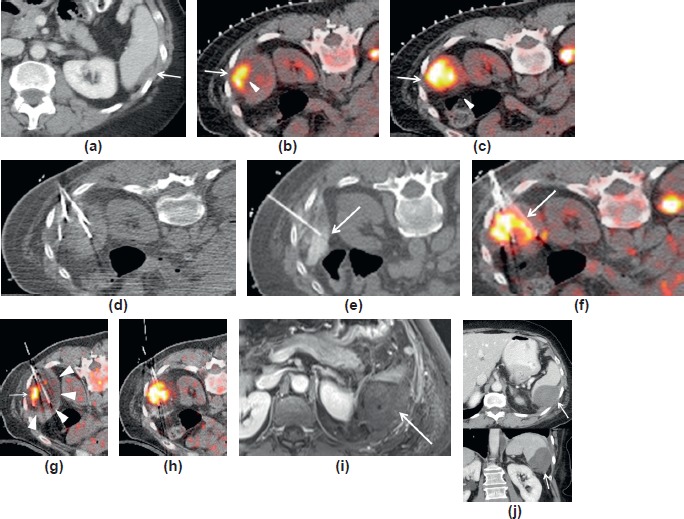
PET/CT-guided cryoablation of perisplenic metastasis in a patient with uterine cancer. (a). Contrast-enhanced CT shows the hypodense mass along the posterolateral border of the spleen (arrow) confirming a solitary peritoneal metastases. (b). Fused PET/CT image acquired using 35 s suspended ventilation prior to probe placement shows the FDG-avid perisplenic peritoneal mass positioned posterior and lateral to the spleen (arrow). The metastatic mass appears partially necrotic in the medial part of the tumour and indents the spleen (arrowheads). Note the radio-opaque skin markers overlying the spleen which are placed in order to help plan the exact coordinates for the placement of the cryoablation probes. (c). Another slice level of the fused planning PET/CT showing large FDG-avid perisplenic peritoneal metastasis (arrow) with its medial surface in close proximity to the left kidney and the anterior surface adjacent to the spenic flexure of the colon (arrowhead). (d). CT fluoroscopy images show placement of the needles and probes into the tumour. (e). CT fluoroscopy shows placement of a 20-G Chiba needle for hydrodisplacement of the splenic flexure of colon with instillation of dilute iodinated contrast into the space between tumour and colonic wall. This manoeuver prevents cryoablation injury of the bowel. (f). Fused PET/CT image shows placement of the hydrodisplacement needle between tumour and bowel wall (arrow). (g). Fused PET/CT at the level of the upper cryoablation probe performed after initial cryoablation shows a hypointense region encompassing the tumour (white arrowheads) which represents the cryoablation iceball. The area of FDG uptake (red arrow) remains unchanged as compared to initial planning PET/CT since FDG does not dissipate from the tumour during or following cryoablation. (h). Fused PET/CT performed towards the end of the cryoablation at the level of lower cryoablation probes shows persistent FDG uptake within the tumour and a hypodense iceball on CT extending beyond the FDG avid tumour. This confirms an adequate ablation margin including and extending beyond the entire tumour. Note the bowel wall is at a significant distant from the cryoablation zone which has been achieved by hydrodisplacement. (i) 3D VIBE breath hold contrast-enhanced MRI shows the hypoenhancing ablation zone (red arrow). No enhancing residual tumour visualised. (j). Follow-up contrast-enhanced CT (axial and coronal) shows the hypoenhancing ablation zone without enhancing residual tumour. There is a small area of reactive left pleural effusion (white arrow) which is expected following ablations adjacent to the diaphragm. The left renal margin adjacent to the ablation zone in the coronal image does not show significant cryoablation-related hypodensity, thus ensuring lack of significant renal parenchymal damage.
